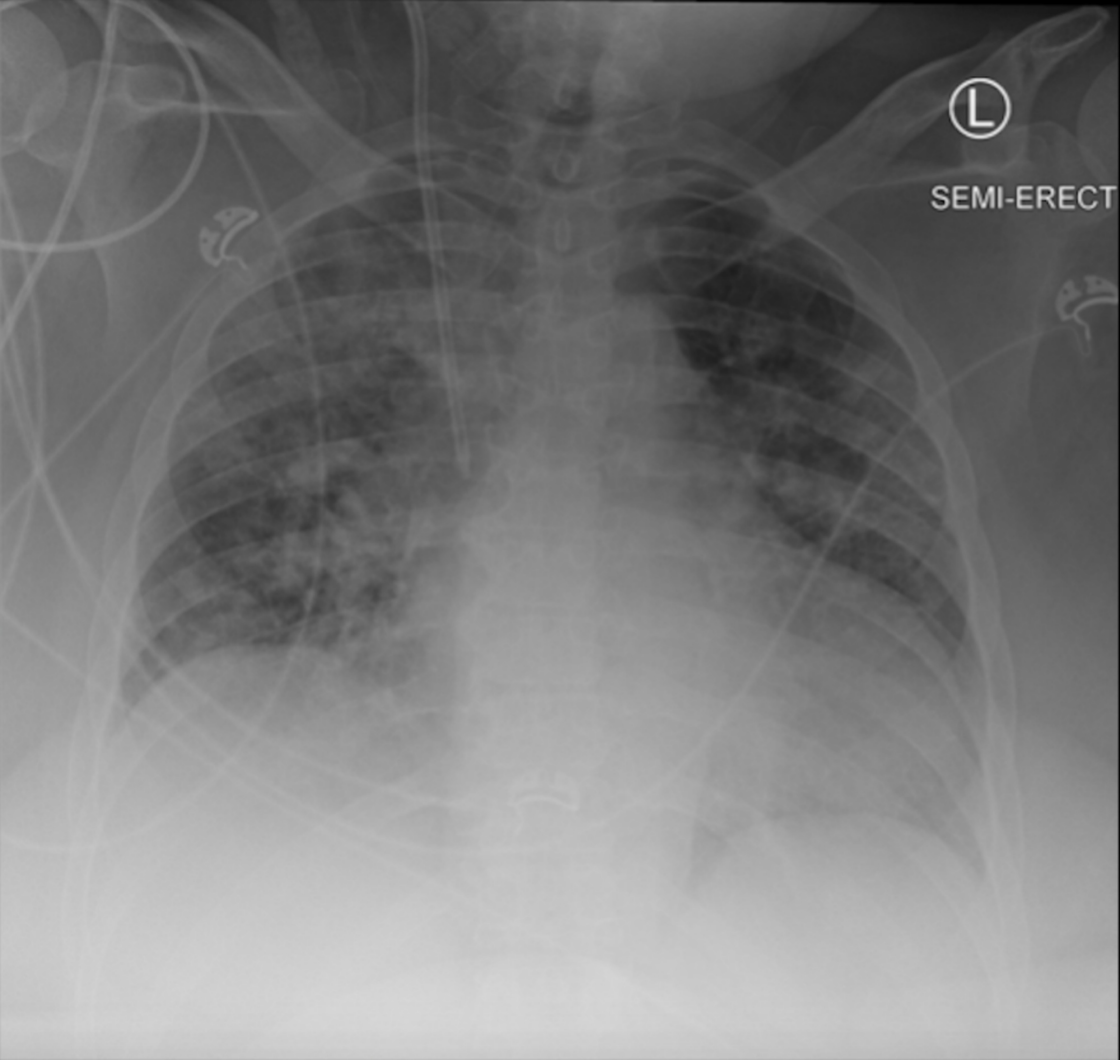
Chest radiograph obtained at our institution on the day of the patient's transfer from the outside hospital
